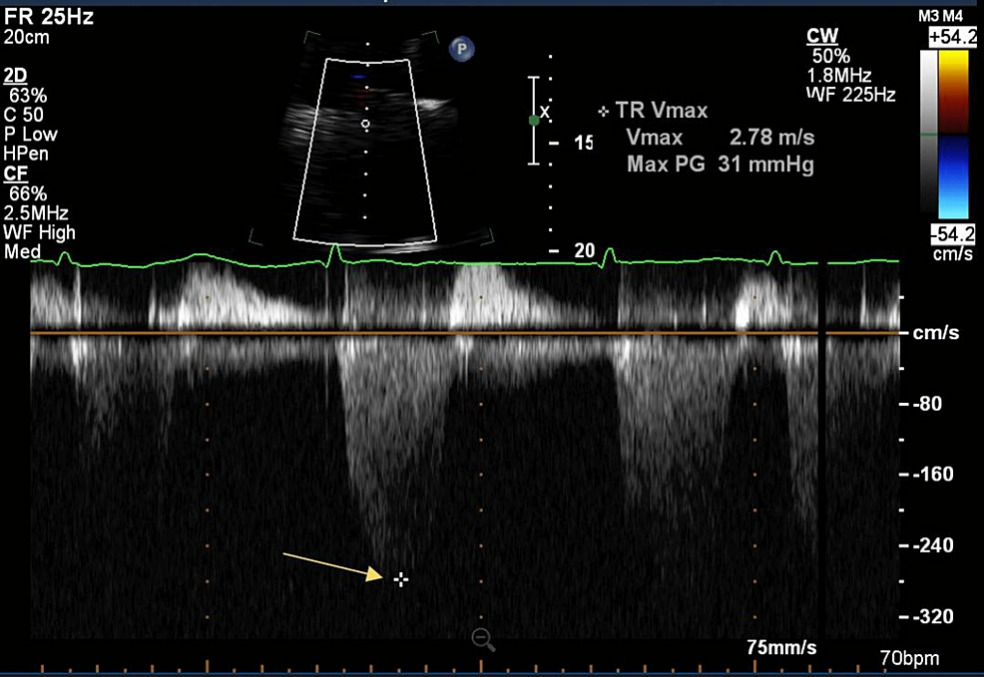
Echocardiography The yellow arrow shows a tricuspid regurgitation velocity maximum of 2.78 m/s
